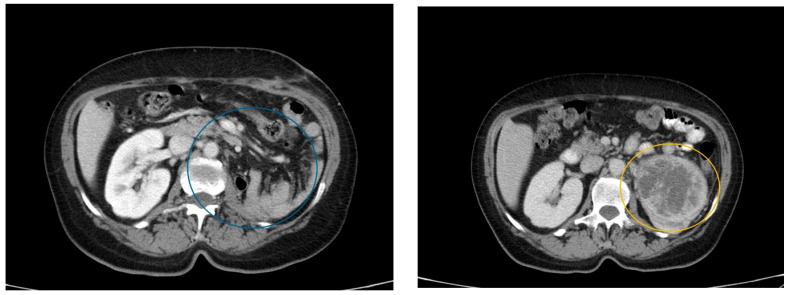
CT scan revealing the tumor formation in the left kidney (marked in blue and yellow circles).
